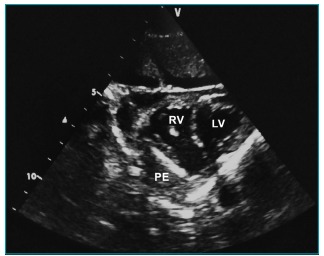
Cardiac ultrasound from a parasternal short axis view. Pericardial effusion with heterogeneous echogenicity and the presence of septations. LV: left ventricle; RV: right ventricle; PE: pericardial effusion.
